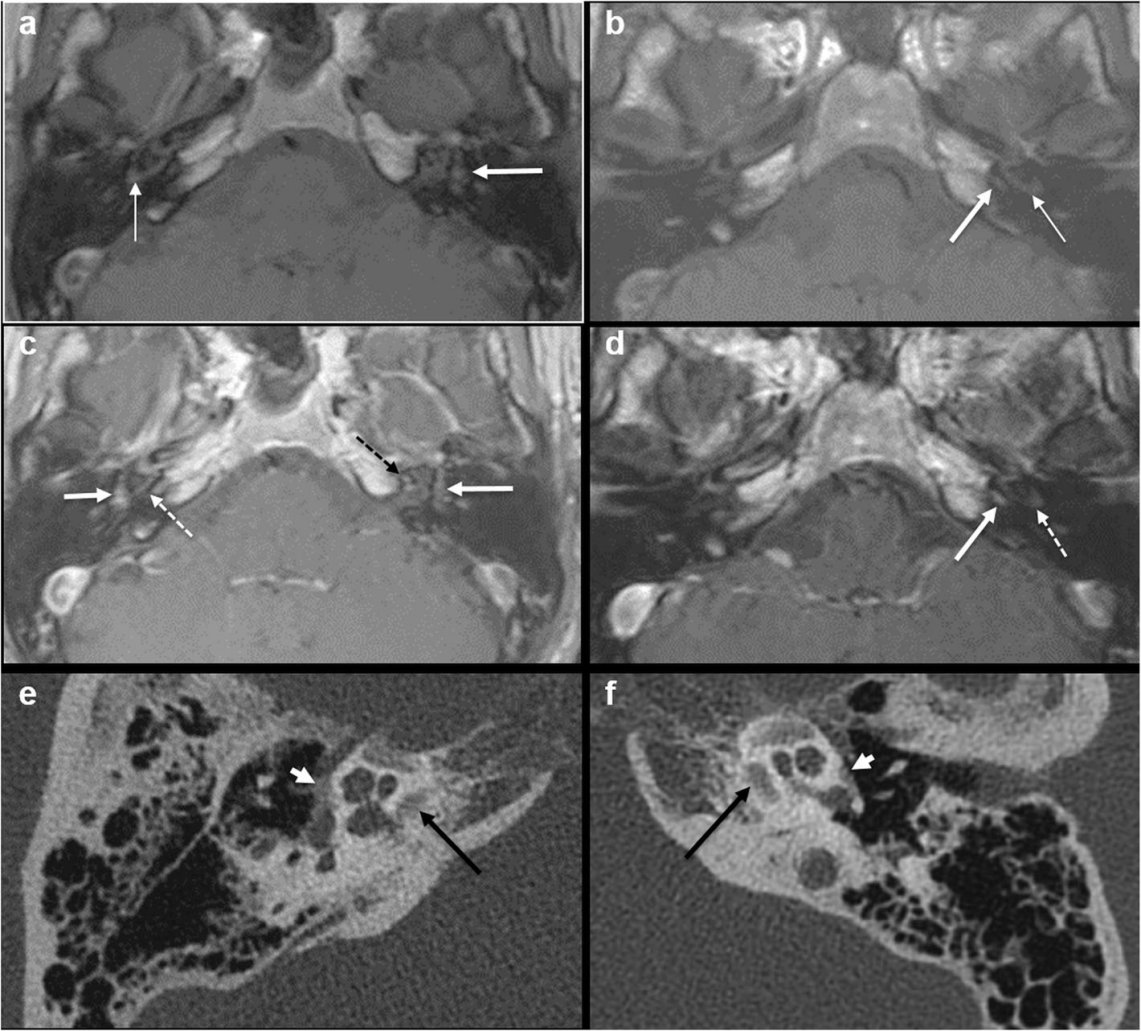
A 46-year-old male patient with right-sided MHL (case 8). Axial T1W MR image (a) shows intermediate T1 signal around the basal turn of the right cochlea (thin arrow) and in the left FA region (thick arrow). Axial T1W MR image at a lower level (b) shows intermediate T1 signal around the basal turn of the left cochlea (thin arrow) and another separate focus of intermediate signal in the left pericochlear region (thick arrow). Contrast-enhanced axial T1W image (c) shows moderate post-contrast enhancement in bilateral FA regions (arrows) and mild enhancement around the basal turns of cochleae (dashed arrows). Contrast-enhanced axial T1W image at a lower level (d) shows mild enhancement around the basal turn of left cochlea (dashed arrow) and another focus of enhancement in the left pericochlear region (arrow). Subsequent axial HRCT images (e, f) confirm bilateral fenestral otosclerotic plaques (short white arrows) and pericochlear plaques (black long arrows)
